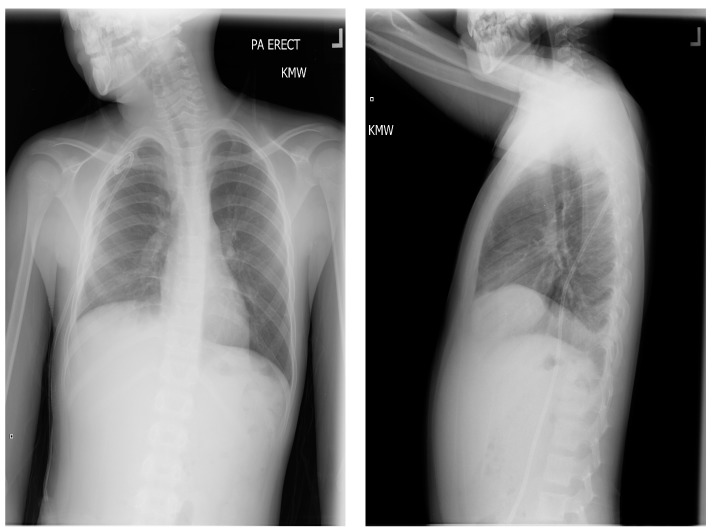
Pre-operative chest X-rays obtained about 1 month after initial presentation, with pigtail chest tube and persistent right chylous effusion (based on imaging and persistent chylous output from chest tube).
